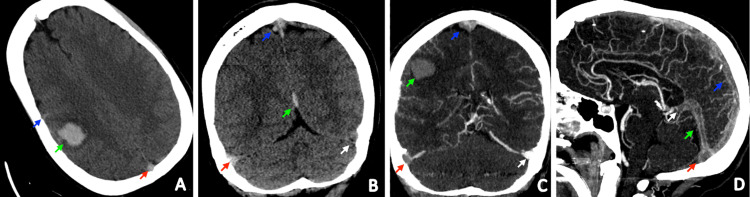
Extensive cerebral venous thrombosis with intracerebral and subarachnoid hemorrhage A. NECT axial image demonstrates a right perirolandic hematoma measuring 2.2 x 1.9 x 2.7 cm (green arrow). There is surrounding vasogenic edema. Adjacent sulcal hyperdensity likely reflects subarachnoid hemorrhage (blue arrow). Hyperdensity is present in the superior sagittal sinus (red arrow). B. Coronal NECT shows hyperdensity in the right transverse sinus (red arrow), superior sagittal sinus (blue arrow), and straight sinus (green arrow). Note that the density of the right transverse sinus is asymmetrically increased relative to the left (white arrow). C. Coronal CTV images demonstrate the right perirolandic parenchymal hematoma (green arrow) with adjacent vasogenic edema. Filling defects are present in the superior sagittal sinus (blue arrow) and right transverse sinus (red arrow) compatible with dural sinus thrombosis. Note that there is normal opacification of the left transverse sinus (white arrow). This explains the asymmetric hyperdensity on the NECT, which was secondary to the thrombus. D. Sagittal CTV images demonstrate filling defects involving the superior sagittal sinus (blue arrow), straight sinus (green arrow), confluence of sinuses (red arrow), and vein of Galen (white arrow), compatible with dural sinus thrombosis CTV: computed tomographic venography; NECT: non-contrast-enhanced computed tomography
